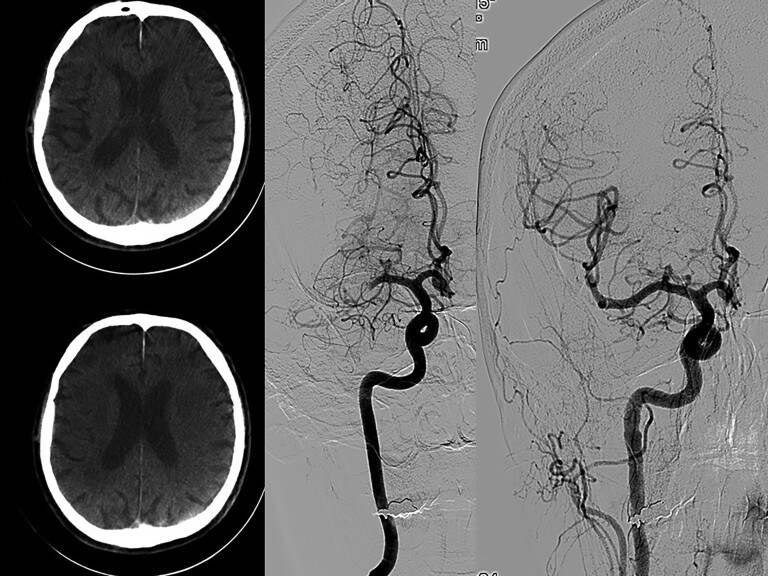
Flat detector computed tomography images (left) with no signs of intracranial bleeding and no clear signs of developed ischaemia. Selective angiogram done immediately after flat detector computed tomography with the same equipment showing middle cerebral artery occlusion (middle) and reopening after thrombectomy (right).
